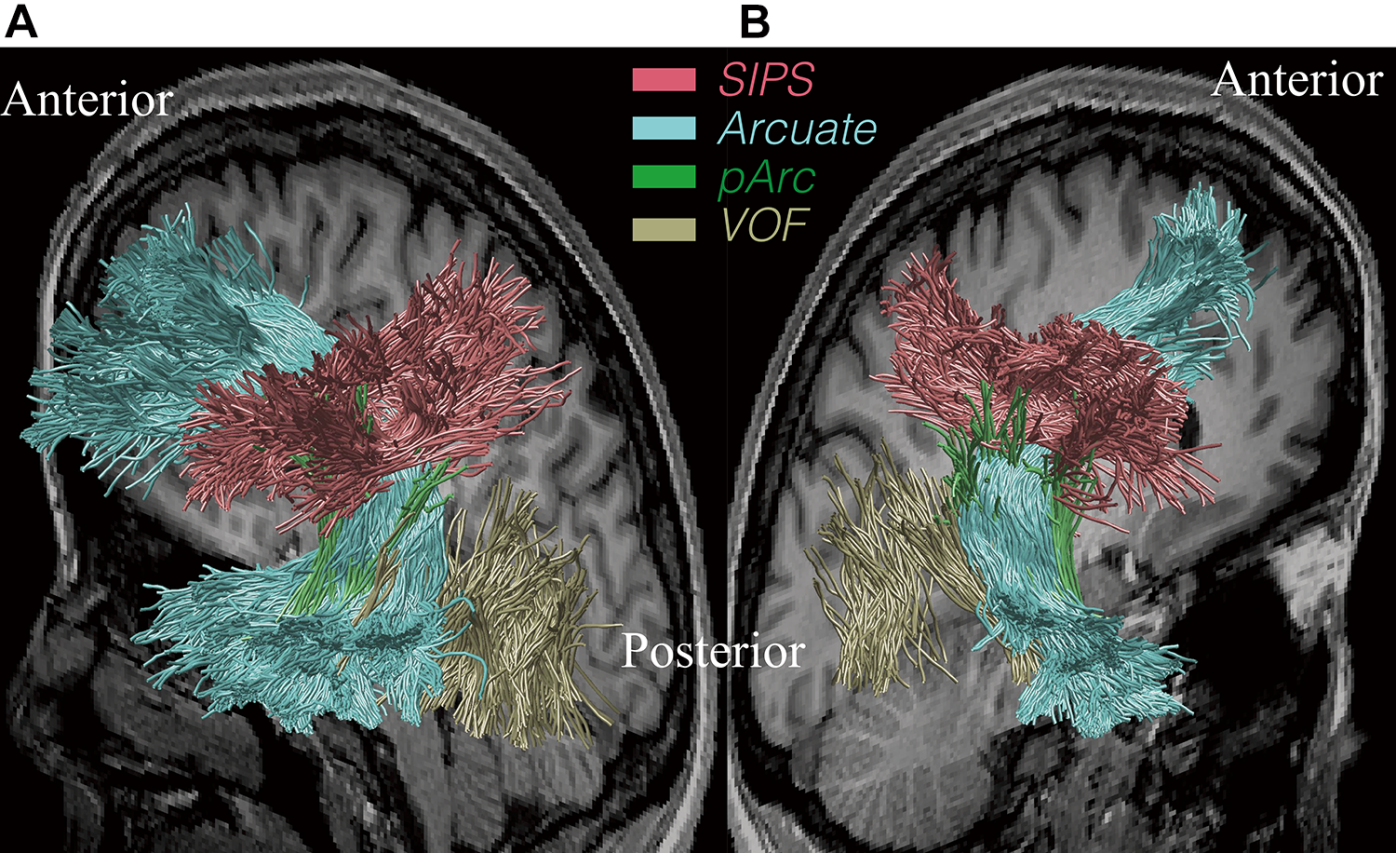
Position of SIPS with respect to other tracts. a Position of SIPS with respect to other tracts in the left hemisphere of one representative subject (S1). The background T1-weighted image is a sagittal slice in the medial portion of the brain. SIPS (*red*) is located superior to the vertical occipital fasciculus (VOF; *yellow*) and posterior arcuate (pArc; *green*). SIPS lies on the superior surface of, and crosses with the arcuate fasciculus (arcuate; *light blue*). b Position of SIPS with respect to other tracts in the right hemisphere in the same subject (S1)
